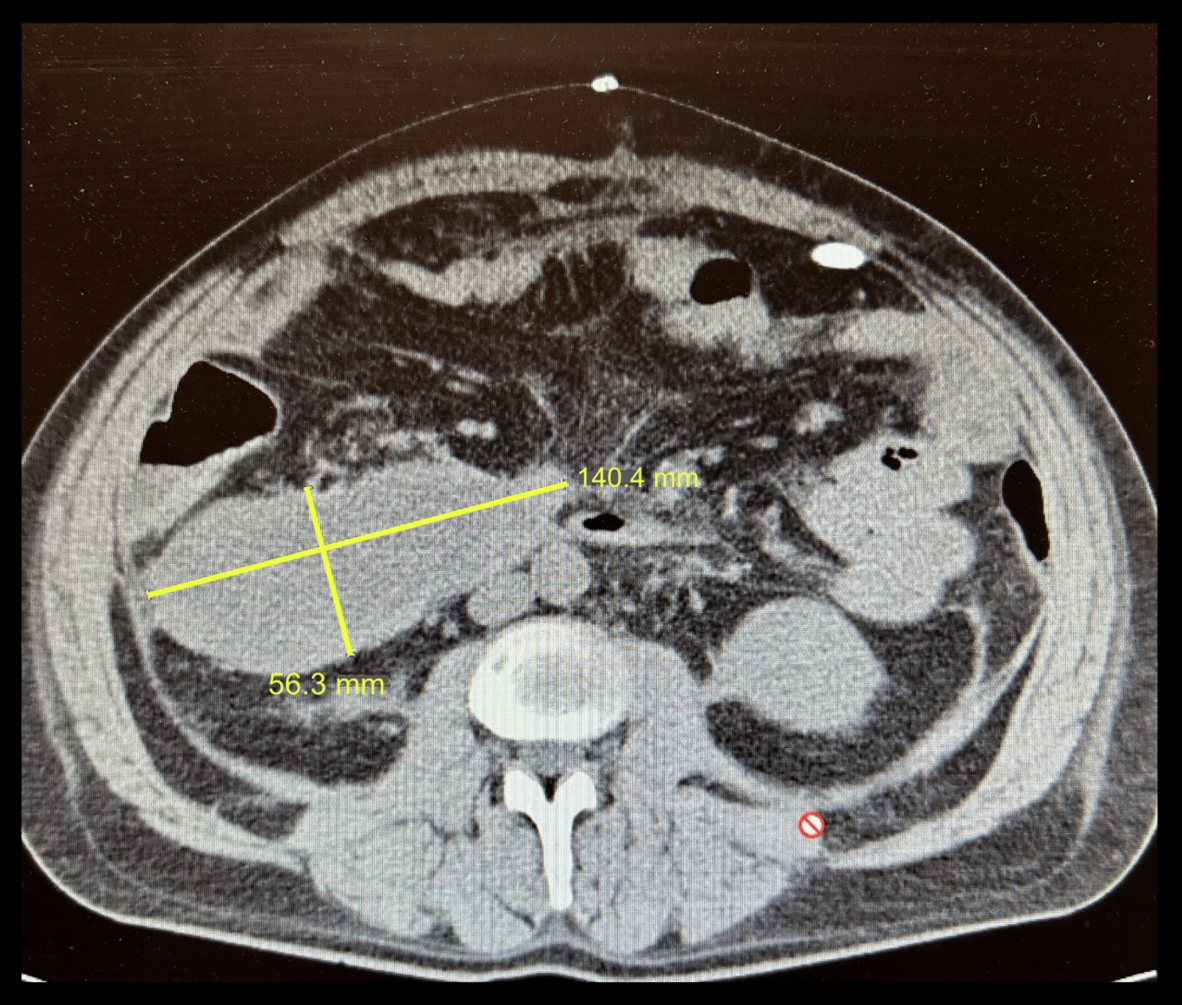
CT abdomen and pelvis demonstrating persistent intraperitoneal hematoma with significant volume reduction from initial presentation and imaging.
